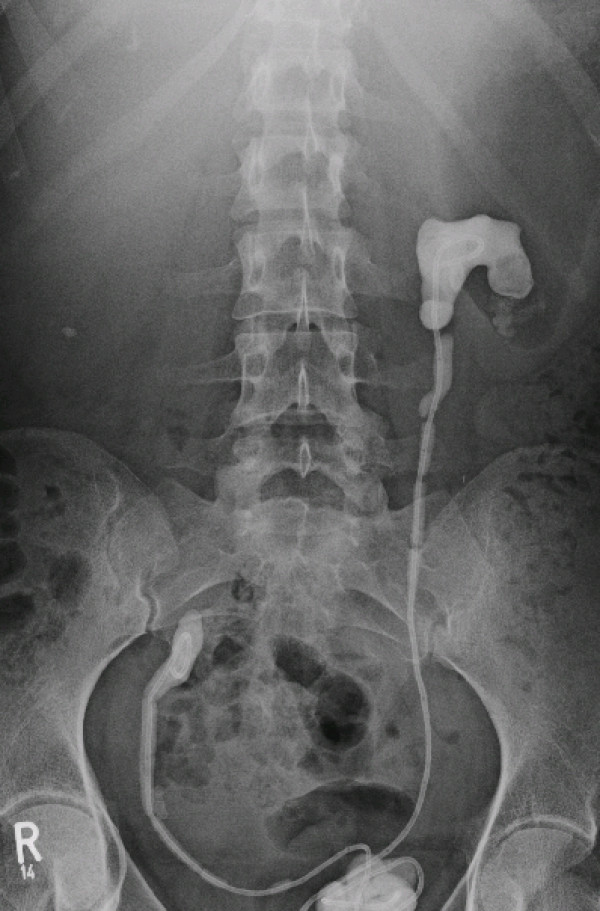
Radiograph before therapy.
